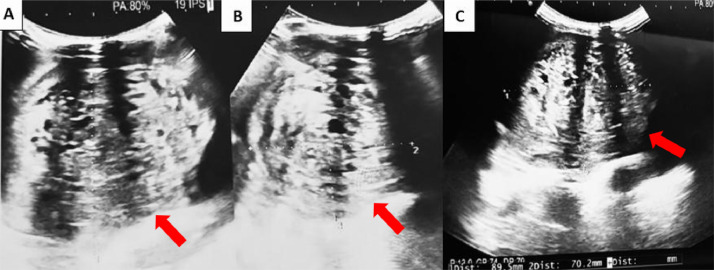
Abdominopelvic ultrasound: Longitudinal (A) and Transverse (B and C) Views. A well-defined, suspicious oval-shaped pelvic mass with regular borders, heterogeneous and hyperechoic, containing several cystic locules (red arrow), measuring 89.5×70.2×66 mm (height x transverse diameter x anteroposterior diameter).
